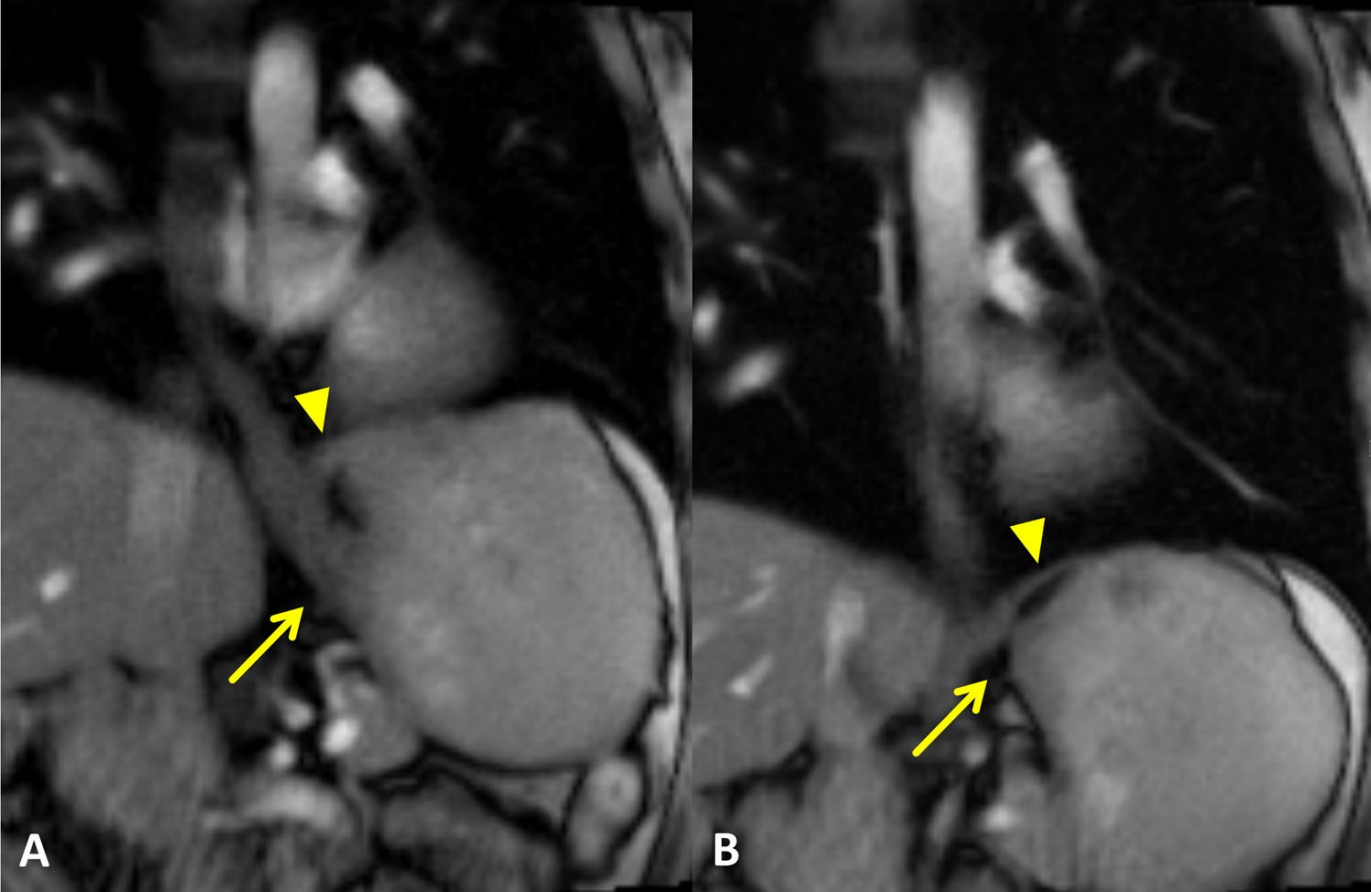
Real-time MRI of the EGJ at rest (A) and during Valsalva (B) in a patient with EGJ type III on HRM. Real-time MRI films at rest revealed a physiologic positioning of the EGJ (arrow) below the diaphragm (arrowhead) (A). Valsalva maneuver resulted in cranialization of the EGJ without herniation through the diaphragm (B). See also supplementary material Video 2
